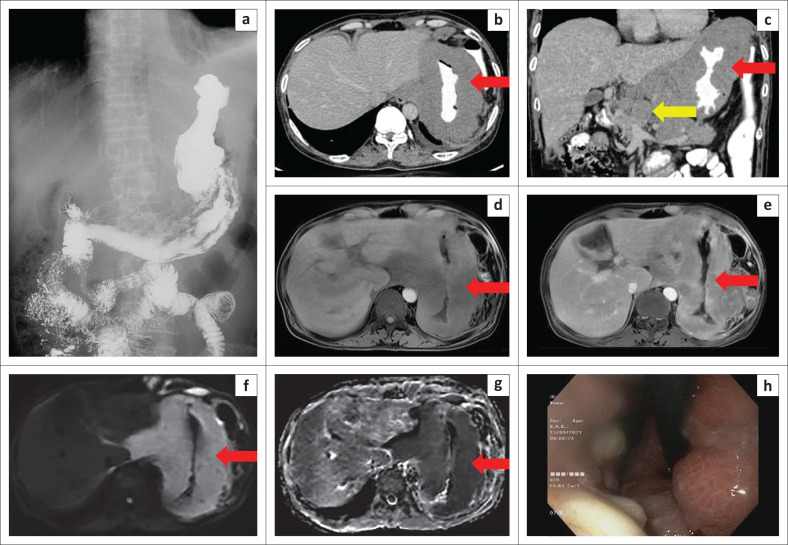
(a) Narrowing of the gastric lumen in the fundus and proximal body. (b, c) Axial and coronal contrast enhanced CT sections reveal significant gastric wall thickening with effacement of the mucosal folds (red arrows) and multiple enlarged discrete lymphnodes (yellow arrow). (d–g) Axial MRI pre- and post-contrast T1 weighted images, diffusion weighted image and ADC images demonstrate the gastric wall thickening with significant restriction (red arrows). (h) Upper GI endoscopy: Nodular thickening in the fundus of the stomach causing luminal stenosis.
